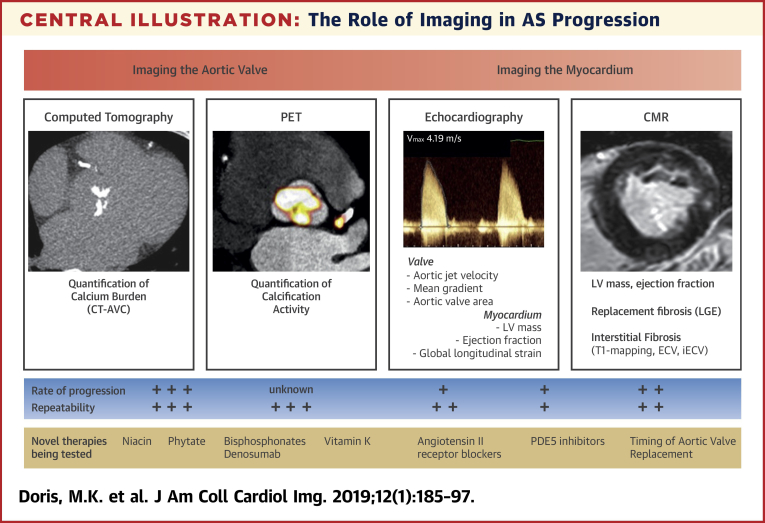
The Role of Imaging in AS Progression Noninvasive imaging provides the ability to directly image the aortic valve and consequent pathophysiological effects on the myocardium. Imaging the valve may be performed by computed tomography (CT) to quantify aortic valve calcification (AVC) load (CT-AVC), positron emission tomography (PET) to measure calcification activity and/or echocardiography to assess hemodynamic severity. The response of the left ventricle (LV) may be assessed by echocardiographic measures of mass, ejection fraction and/or strain, whereas cardiac magnetic resonance (CMR) offers additional quantification of fibrosis. The ability of these techniques to detect therapeutic efficacy depends on the scan-rescan repeatability of the test and the rate of change of the parameter being measured. These attributes are being exploited in a number of ongoing studies to investigate novel therapies for aortic stenosis. AS = aortic stenosis; ECV = extracellular volume; iECV = indexed extracellular volume; LGE = late gadolinium enhancement; PDE5 = phosphodiesterase type 5.
